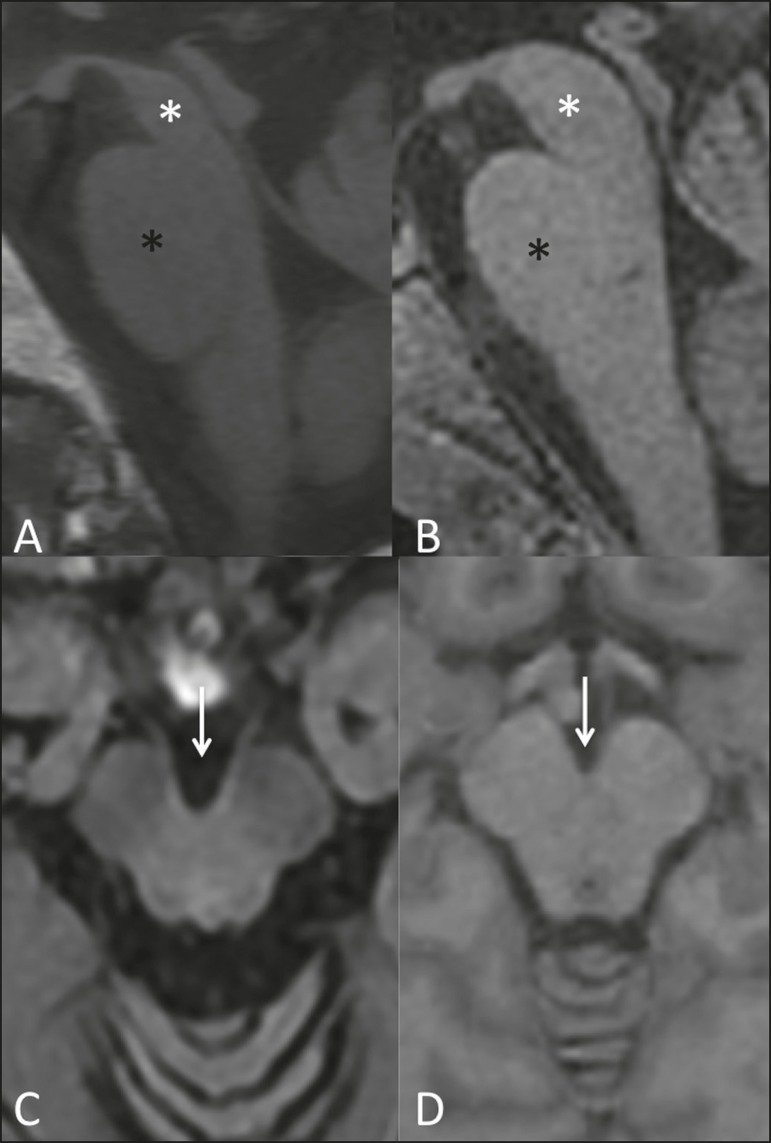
Conventional MRI findings in progressive supranuclear palsy. In a
